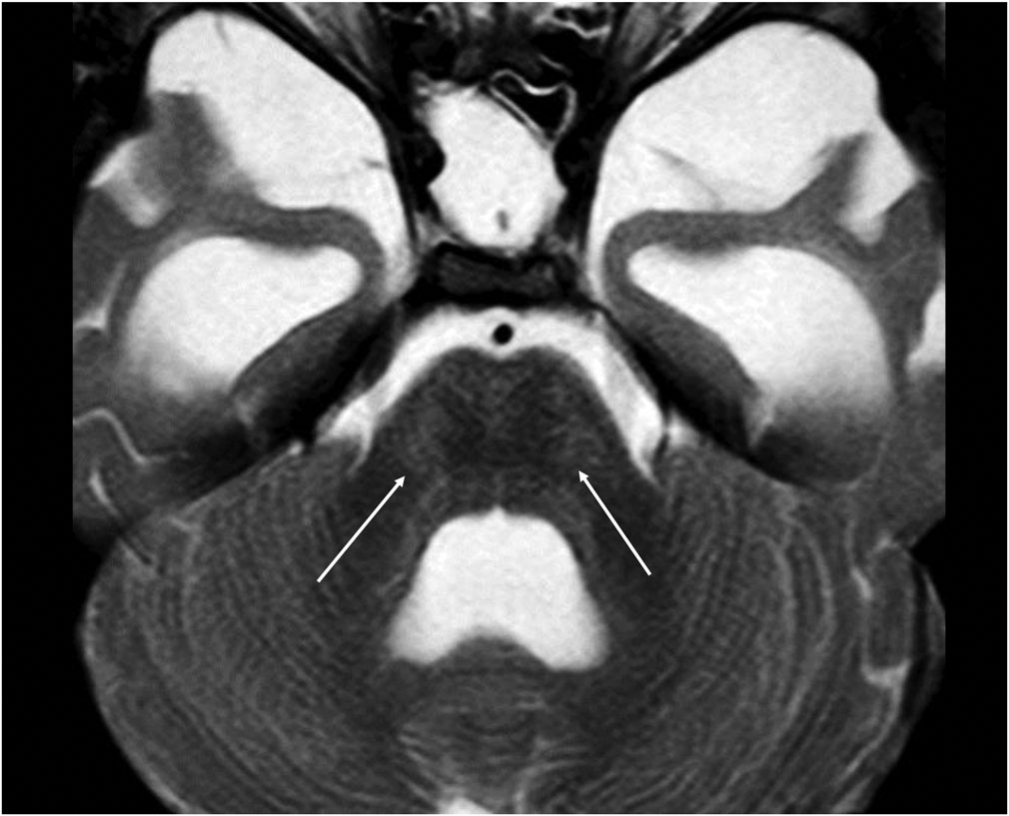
MPS IIA in a 7‐year‐old boy. Axial T2‐weighted image shows areas of slight T2‐FLAIR hyperintensity in the pons, characterizing the presence of white matter lesions. FLAIR, fluid‐attenuated inversion recovery; MPS, mucopolysaccharidoses
